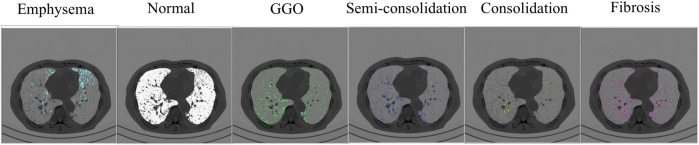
The severity of parenchymal abnormalities in subjects is determined by CT Hounsfield units. The visualization demonstrates multiple lung states, spanning from emphysematous regions to normal parenchyma, areas of semi-consolidation and consolidation, and fibrotic changes.
